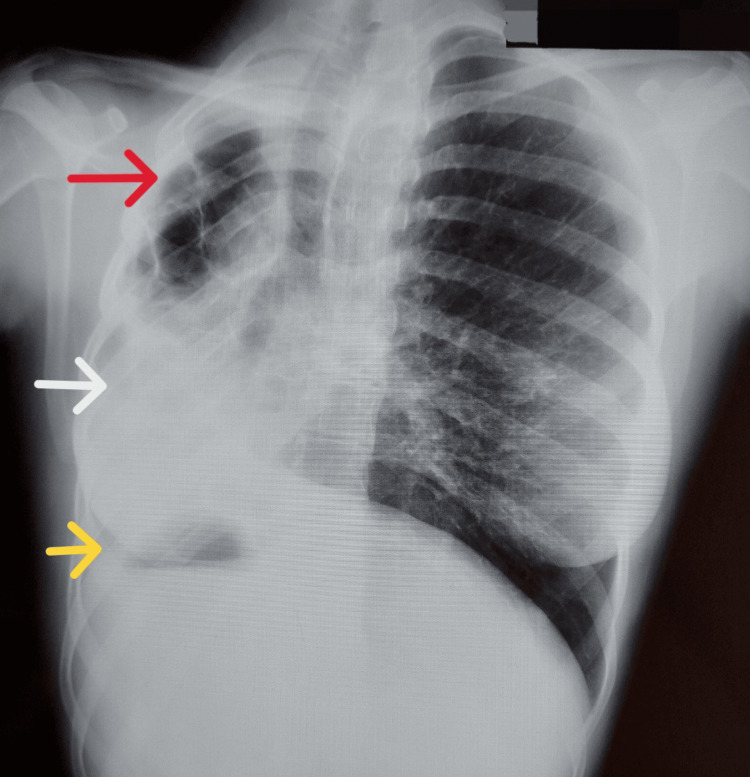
Chest X-ray The chest X-ray showed significant lung damage (red arrow) with rightward deviation of the trachea and cardiac silhouette (white arrow), with left lung hyperinflation. The stomach bubble is on the right side (yellow arrow), suggesting situs inversus.
